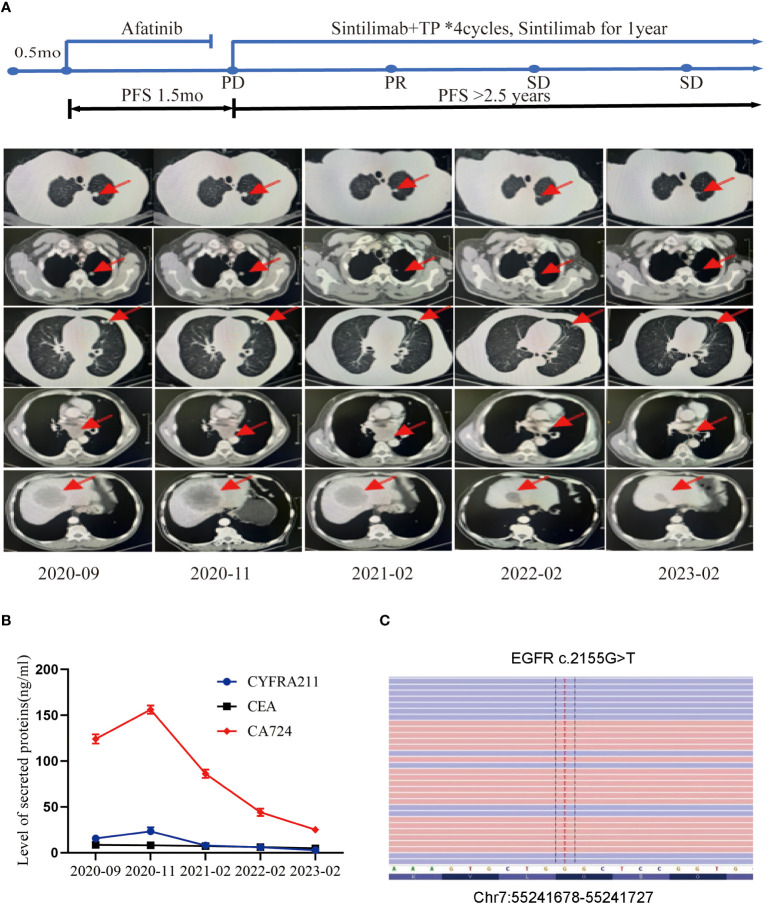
Tumor progression of the patient during the treatments. (A) The timeline of the therapies and imaging results of the computed tomography (CT) can during diagnosis and treatment at different time points. (B) The levels of secreted protein during treatment at different time points. (C) The site of EGFR mutation.
